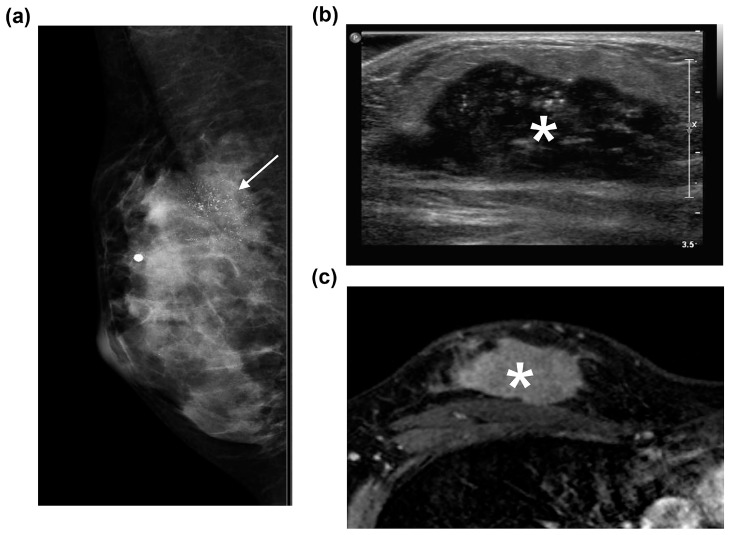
Imaging findings of ER+/PR+/HER2− invasive breast cancer of no special type with high Ki-67 expression in the right breast of a 17-year-old female. (a) Right mediolateral oblique view of the mammography showing a 5 cm, irregular, not circumscribed isodense mass with a segmental distribution, as well as fine, linear-branching suspicious calcifications (arrow) in the upper breast. (b) B-mode ultrasound image showing a large, irregular, not circumscribed, hypoechoic mass (asterisk) with an echogenic rind. (c) Axial contrast-enhanced fat-suppressed T1-weighted magnetic resonance imaging showing an irregular, non-circumscribed, heterogeneous, rim-enhancing mass (asterisk) with washout kinetics in the right upper outer quadrant of the breast. The patient underwent breast-conserving surgery with axillary lymph node dissection after two years of palliative chemotherapy for bone metastasis of the thoracolumbar spine at the time of diagnosis, followed by additional radiotherapy with hormone therapy. Three years after surgery, pleural metastasis with hilar lymph node metastasis was observed, and the patient died two years after chemotherapy. ER: estrogen receptor; PR: progesterone receptor; HER2: human epidermal growth factor receptor 2.
